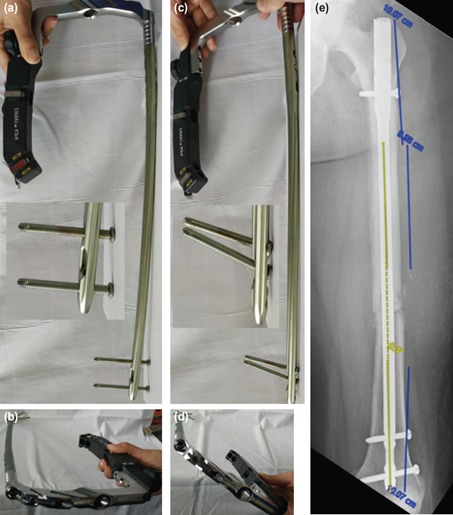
A2FN assembled to insertion handle, coronal alignments of the nail in relation to distal screws holes and insertion handle were shown. (a) When distal static screws holes are in true lateral plane, the nail is valgus, and (b) the insertion handle is horizontal. (c) When distal dynamic screw hole is in true lateral plane, the nail is straight, and (d) the insertion handle is anteverted (approximately 30°). (e) An example of femoral radiograph with A2FN shows that the lower two third of nail is straight when distal dynamic hole is well visualised.
